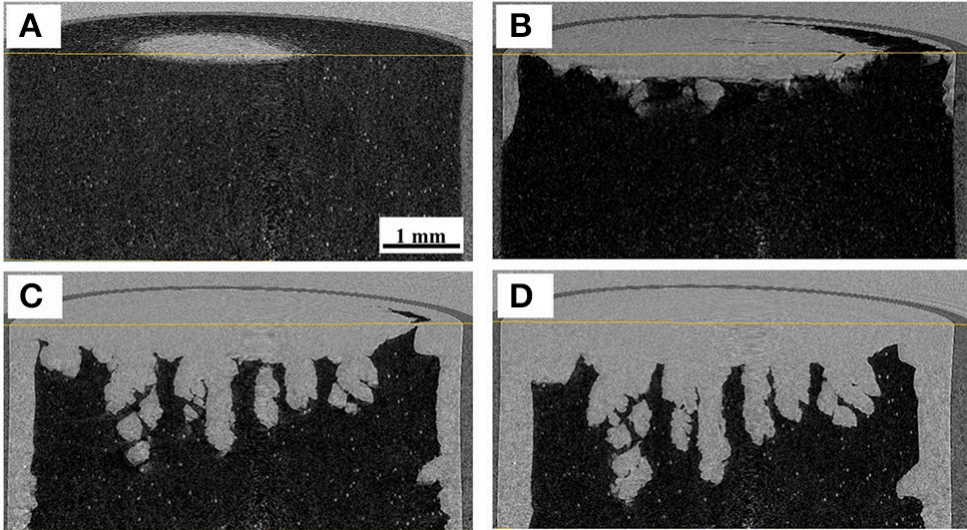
X-ray CT images during ice sublimation, for samples dried for (A) 0, (B) 600, (C) 1,200, and (D) 1,800 s.
